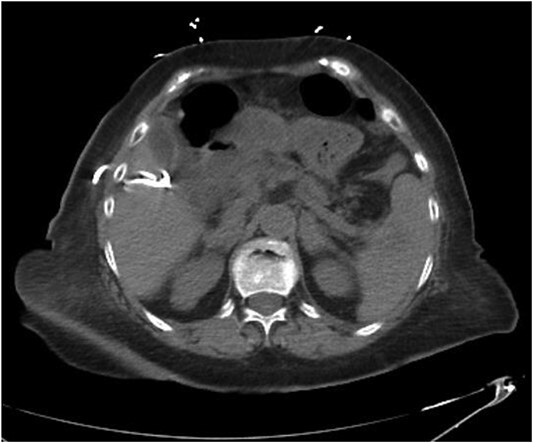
CT abdomen and pelvis demonstrating positioning of PCT.
